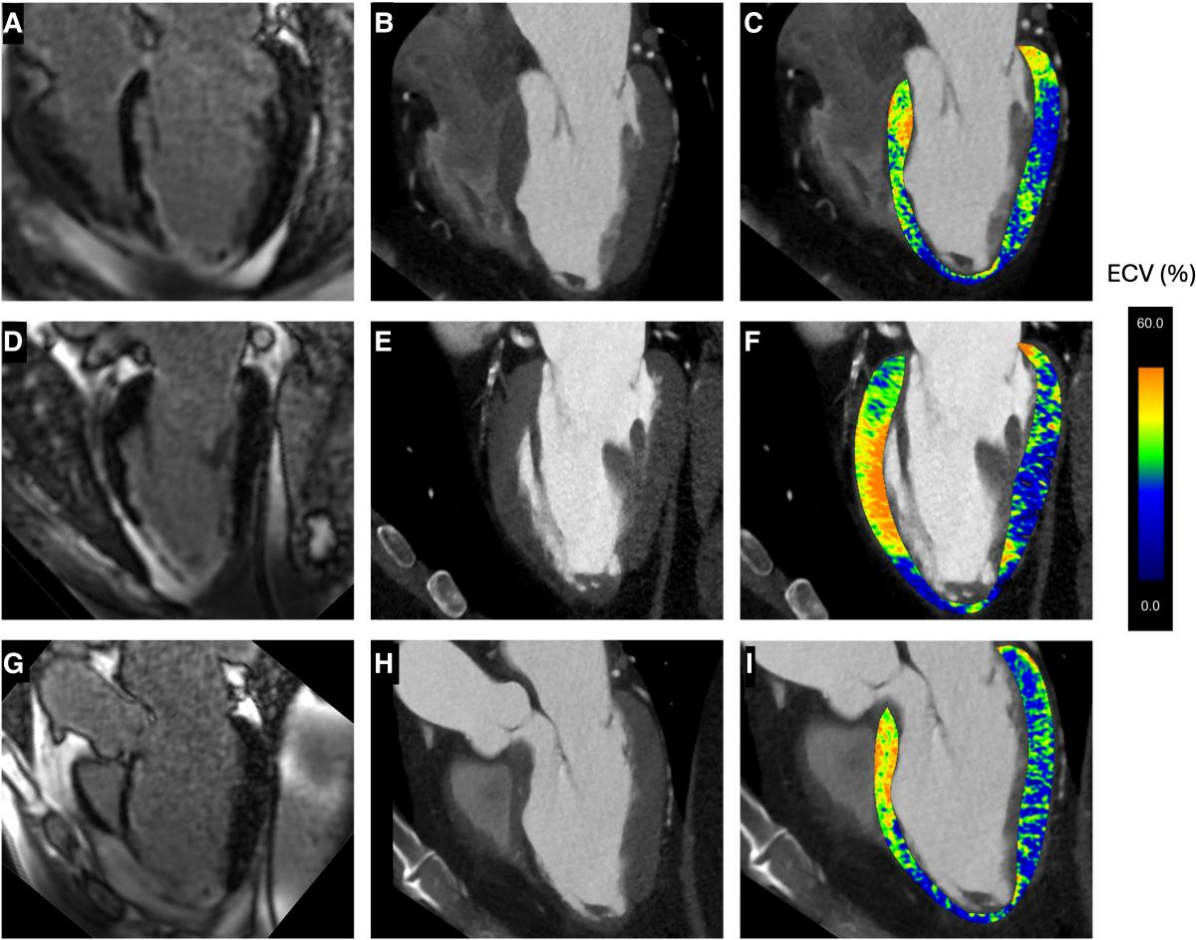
Multimodal imaging of lipomatous metaplasia (LM) – long axis planes. Panels A–I are arranged in rows corresponding to four- (A–C), two- (D–F), and three-chamber apical views (G–I) of the left ventricle. The left column (*A*, D, *G*) CMR images of late gadolinium enhancement, the middle column (*B*, E, *H*) shows corresponding CT angiography images, and the right column (*C*, F, *I*) displays colour-coded ECV values overlayed on the CT image. Abbreviations: CMR – Cardiac Magnetic Resonance, CT – Computed Tomography, ECV – Extracellular Volume.
